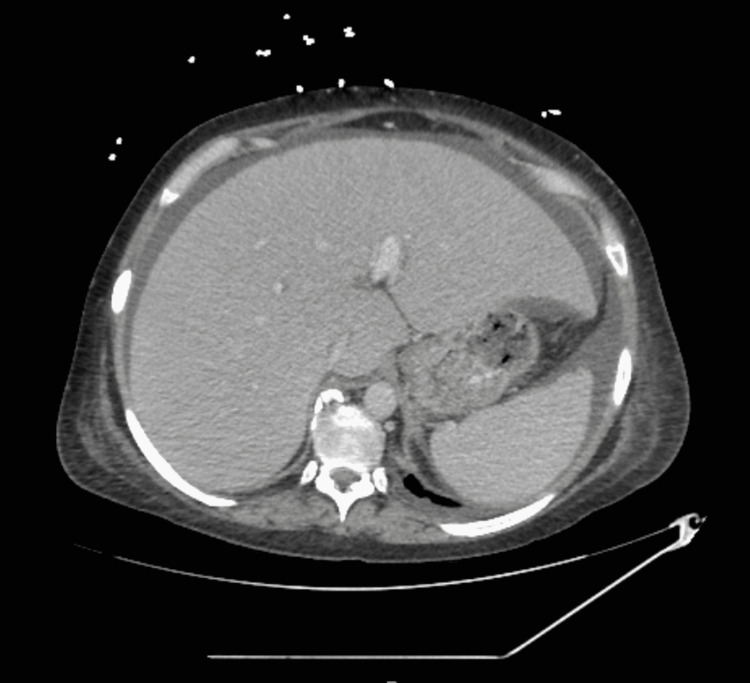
CT of the abdomen/pelvis with IV contrast (cross-sectional view)
